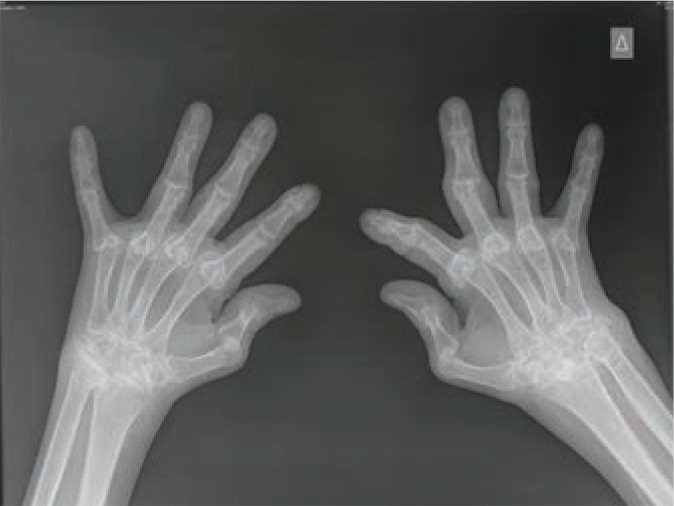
Hand x-rays. Note the severe erosive changes and subluxations of the MCP joints bilaterally, fusion of the carpal bones, and severe osteopenia.
